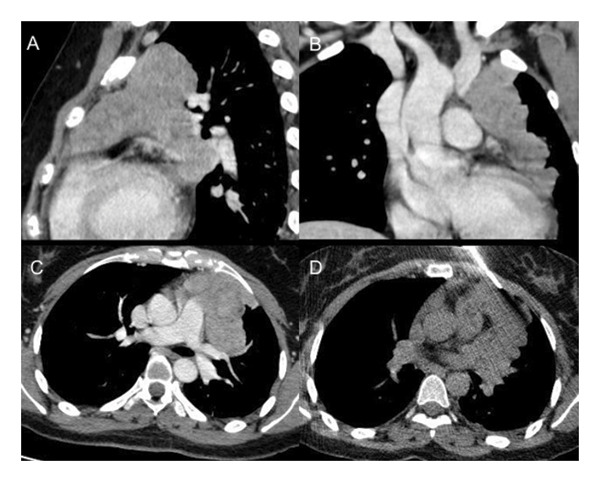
Chest computed tomography with and without contrast. (A) Sagittal, (B) coronal, and (C) axial views demonstrate a lobulated, solid, enhancing mass in the anterior mediastinum measuring 8.7 × 6.0 × 8.8 cm. Anteriorly, it is in contact with the chest wall. Posteriorly, the mass extends into the posterior mediastinum and abuts the left superior pulmonary vein, main pulmonary artery, and left atrial appendage. Superiorly, there is mass effect and evidence of invasion into the left brachiocephalic vein. A left pleural drop metastasis is also noted. (D) Axial view from a noncontrast CT performed during core needle biopsy.
